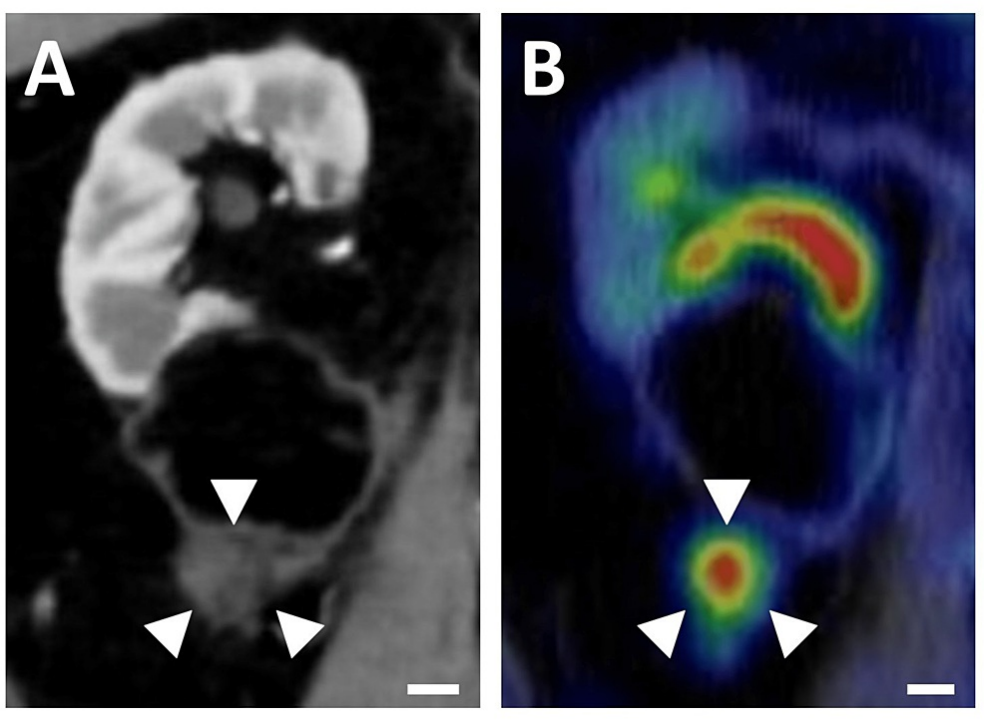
Nodular lesion in the perirenal fat with FDG-PET positivity. (A) Contrast-enhanced CT scan shows a retroperitoneal 2-cm nodule adjacent to the right perirenal fat (white arrowheads). Scale bar, 1 cm. (B) PET-CT exhibited an uptake (SUV-max 5.5) in the same nodule (white arrowheads). Scale bar, 1 cm.
